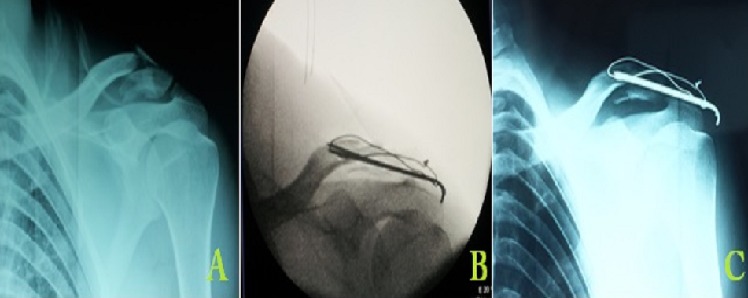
Homme de 28 ans avec une fracture type II de Neer ayant bénéficié d'un brochage tranacromiale renforcé par un hauban au fils d'acier. (A) radiographie préopératoire montrant une fracture type II de Neer. (B) contrôle per opératoire montrant la réduction de la fracture. (C) contrôle radiographique à 4 mois au moment de l'ablation de matériel
